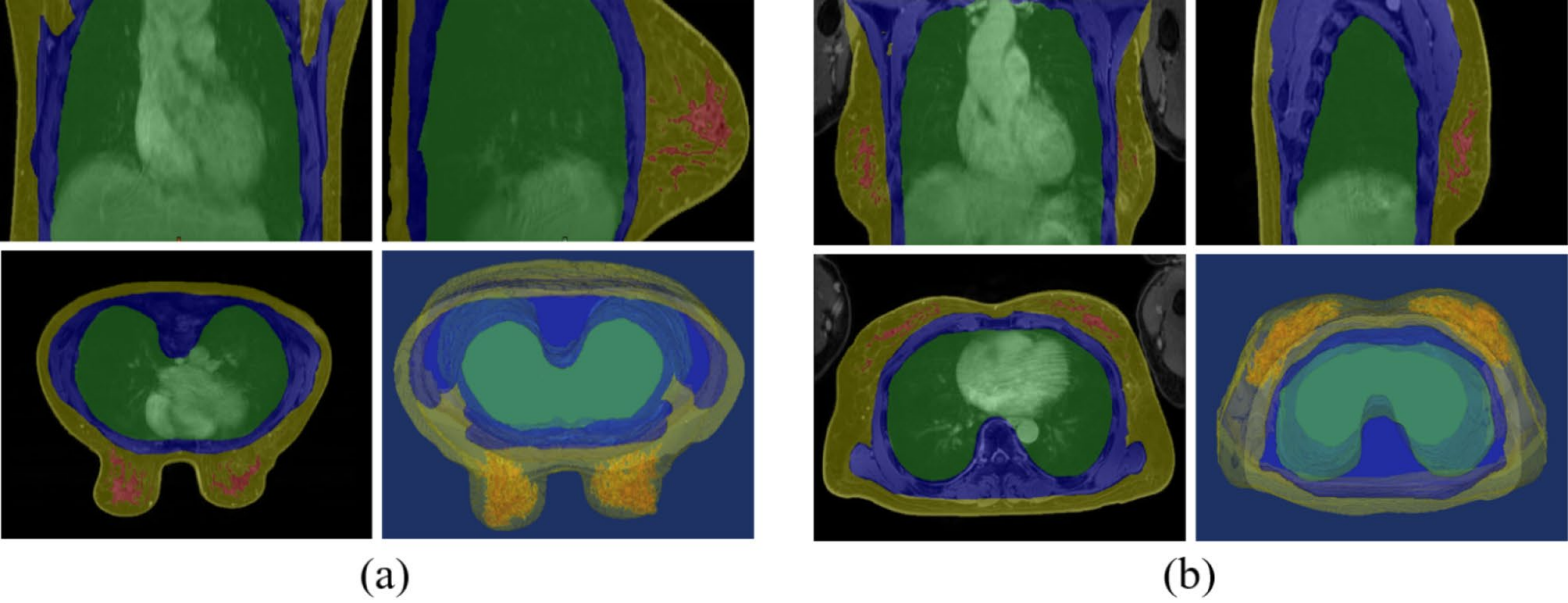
Typical multi-planner reformatted views. (a) prone MRI, and (b) supine MRI. (Left to right in the upper row: coronal and sagittal views; in the lower row, axial and 3D visualization views) (Colors: green: lungs and heart; blue, muscles and bones; red: parenchyma with cancer; yellow: skin and fat without parenchyma with cancer).
